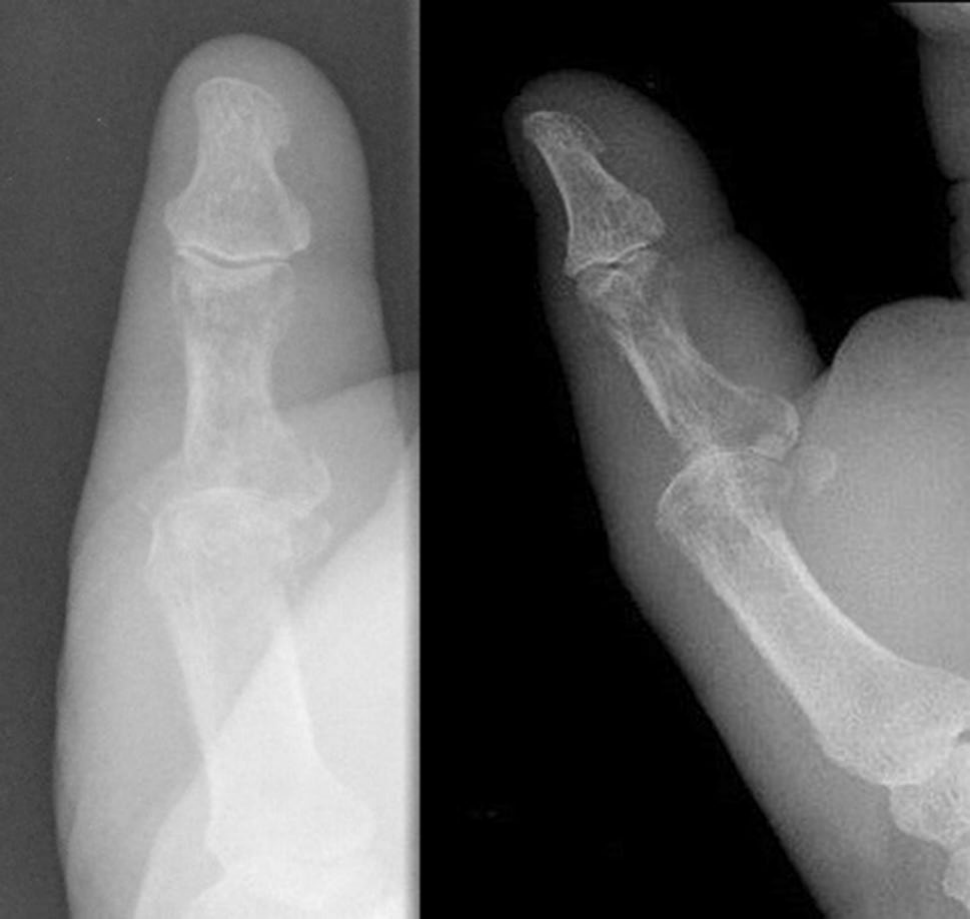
X-ray showing osteomyelitis of the proximal phalanx of the right thumb
